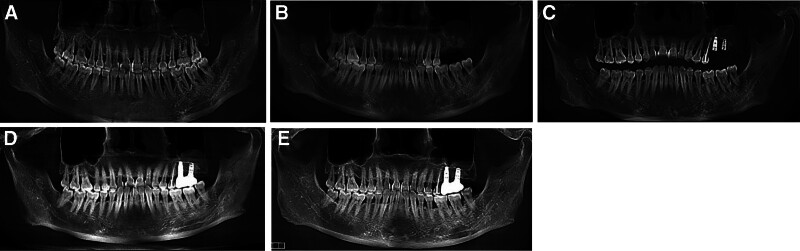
Panoramic radiograph. (A) Pretreatment. (B) Six months after periodontal surgery. (C) One year after periodontal surgery. (D) Three year after periodontal surgery. (E) Five year after periodontal surgery.
